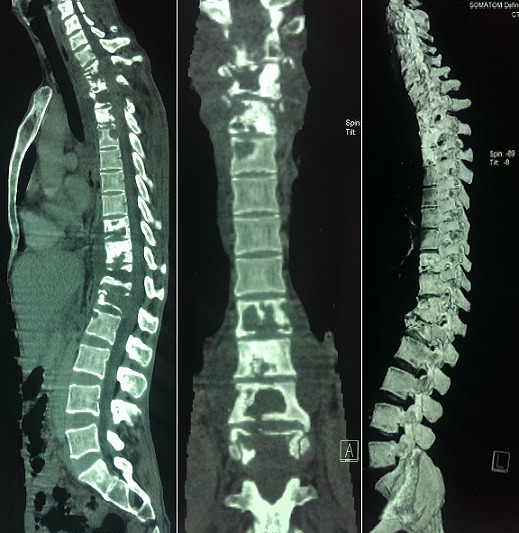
A) spinal CT scan on sagittal section (bone window) objective two non contiguous locations, cervicodorsal and thoracolumbar lesions with typical mirroring invasion of the vertebral plate; B) coronal section (bone window): note the normal aspect of the vertebral bodies between the two locations; C) 3D reconstruction: cervicodorsal (C7 to T4) and thoracolumbar (D10 to L1) achievement
